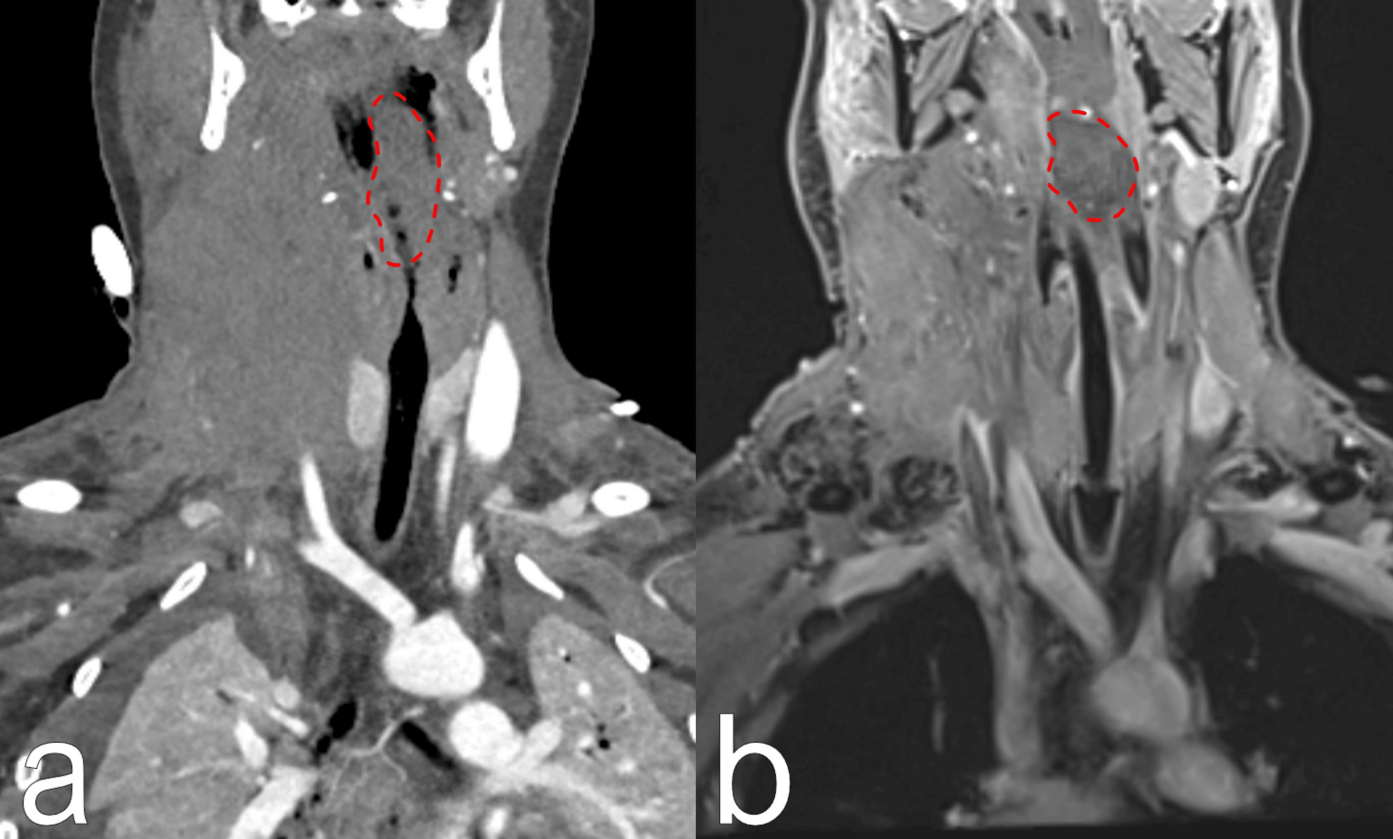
Patient's MRI and CT scans a) The CT scan on the day of complication; b) The MRI after decannulation from extracorporeal membrane oxygenation (ECMO)
